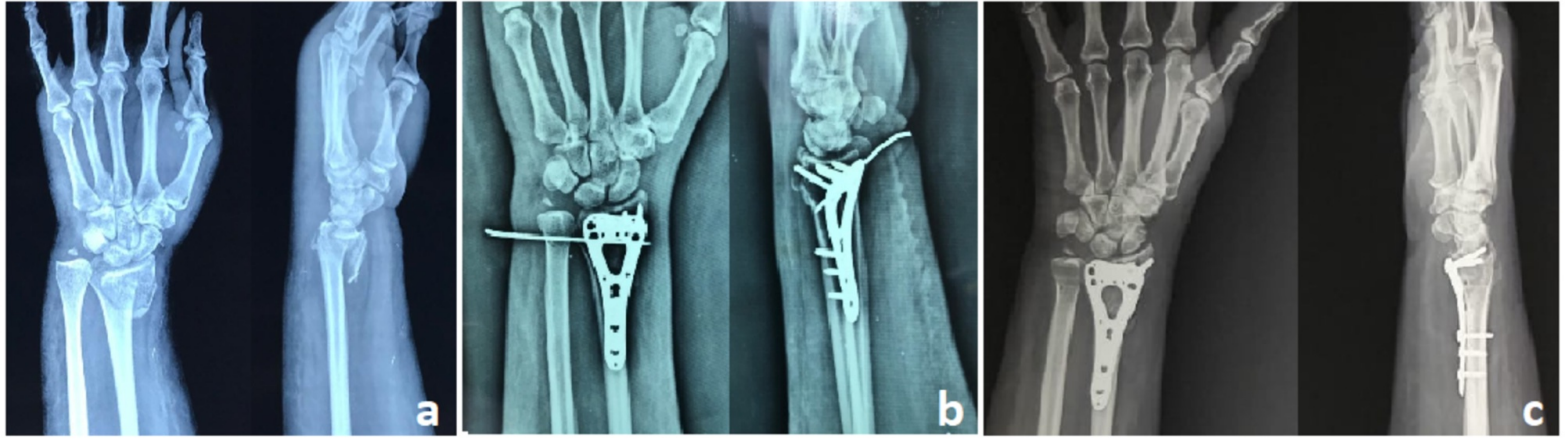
A: Preoperative radiograph showing intraarticular distal radius and ulnar styloid fracture; B: Postoperative radiograph with the volar locking plate and DRUJ K wire in situ; C: Six-month follow-up radiograph showing radiological union DRUJ: Distal radioulnar joint; K wire: Kirschner wires
